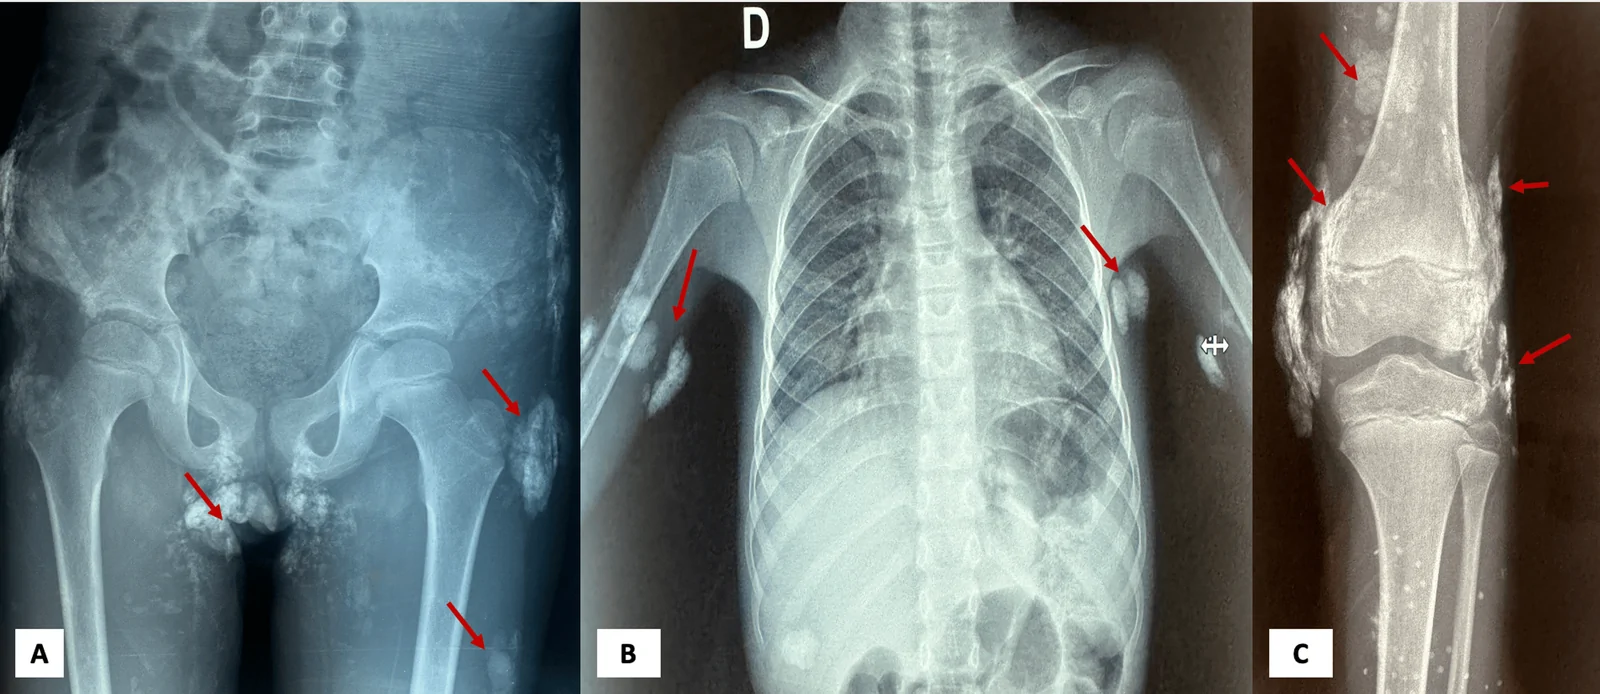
Radiographic appearance of calcinosis cutis. (A) Pelvic radiograph showing extensive, irregular, and lobulated radiopaque calcified deposits within the soft tissues of the gluteal region. (B) Trunk radiograph demonstrating multiple diffuse and multifocal radiopaque calcifications distributed throughout the subcutaneous soft tissues. (C) Knee radiograph showing periarticular soft tissue calcifications with a multilobulated appearance, without evidence of underlying bone involvement. Red arrows indicate the calcinosis lesions in all panels.
